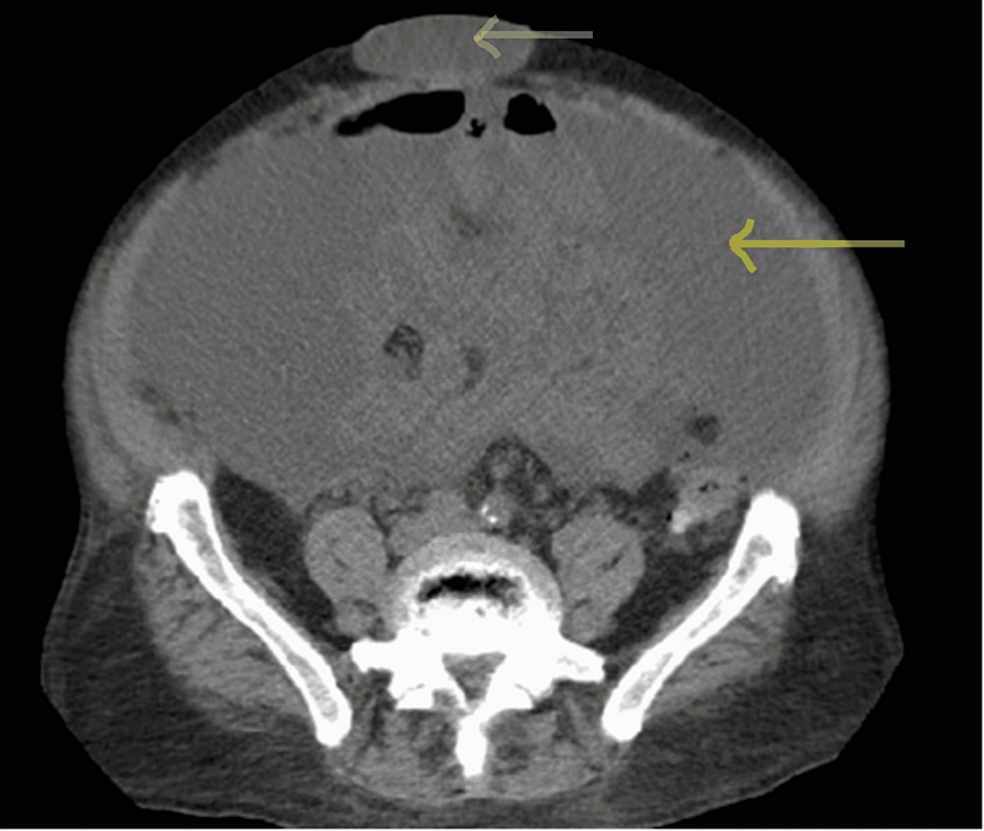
CT scan of the abdomen with IV contrast after paracentesis CT scan of the abdomen with IV contrast after abdominal paracentesis showing improved abdominal ascites (yellow arrow), and a new bowel loop inside the umbilical hernia (red arrow).
